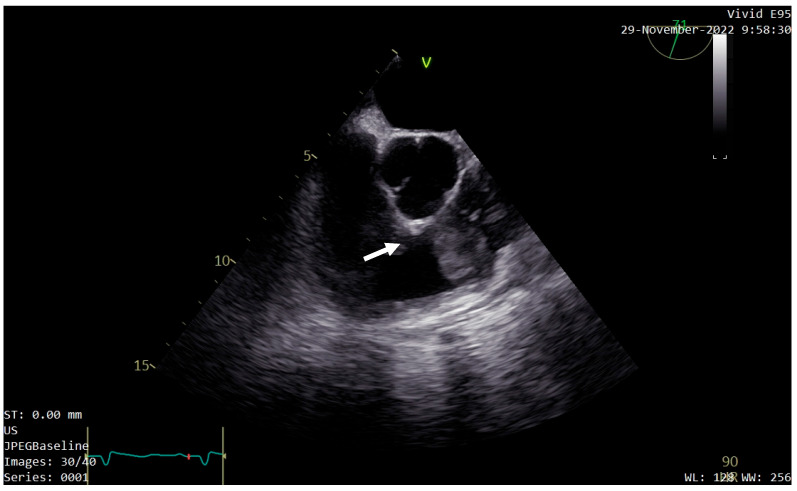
Transesophageal echocardiography (TEE) confirming the location, size, and shape of the tumor (white arrow).
